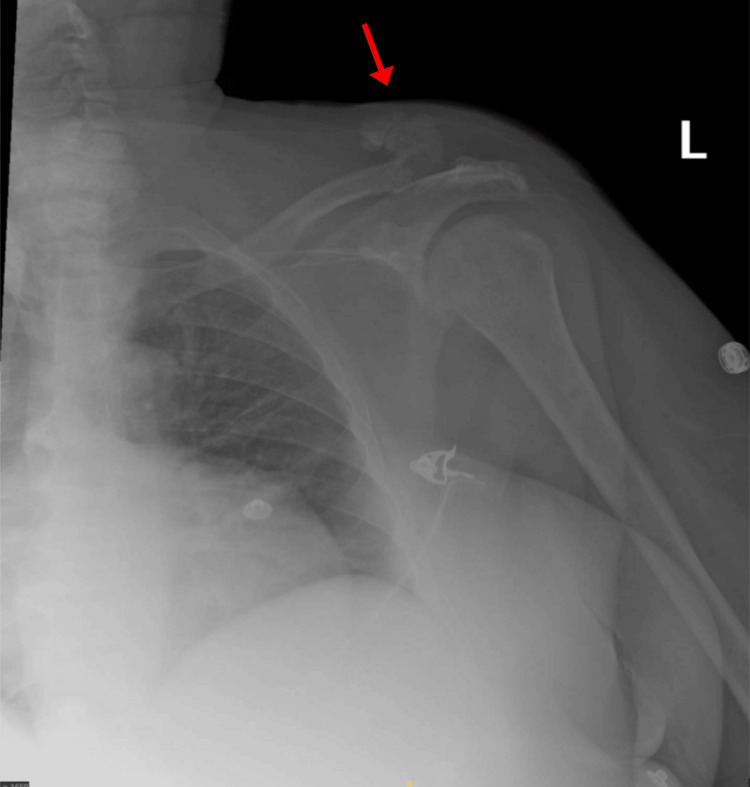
X-ray demonstrating left acromioclavicular joint involvement.
